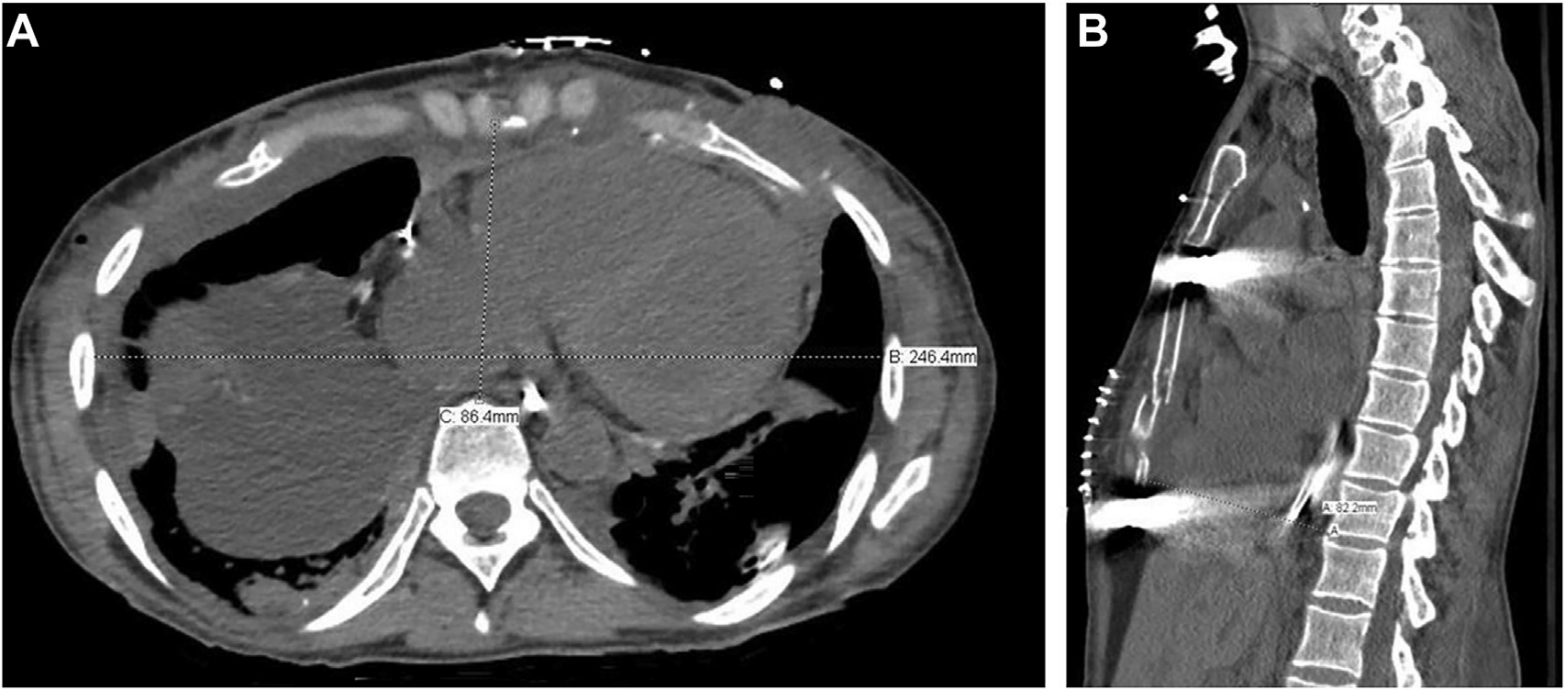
Postoperative computed tomographic scan after bilateral lung transplantation and Nuss bar placement shows improved thoracic configuration. (A) Axial view. (B) Sagittal view.
